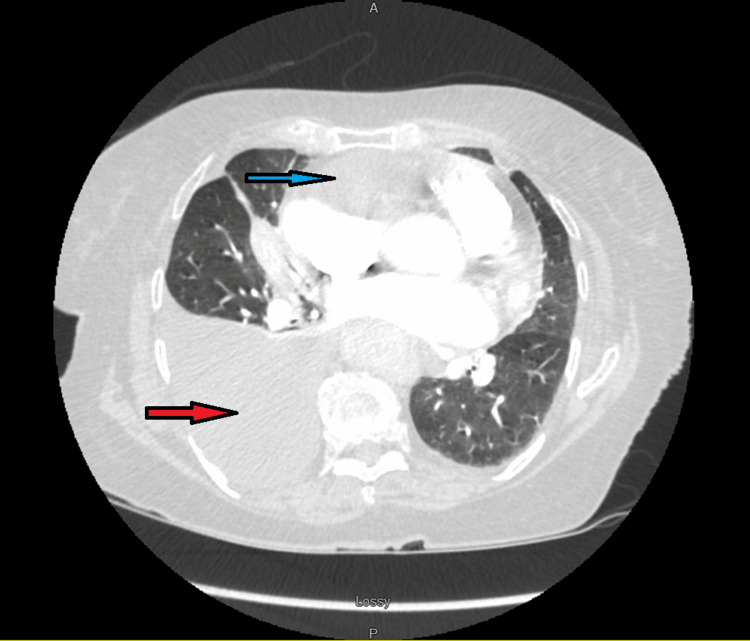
CT angiogram of the chest showing the large mediastinal mass (blue arrow) and right-sided pleural effusion (red arrow).
